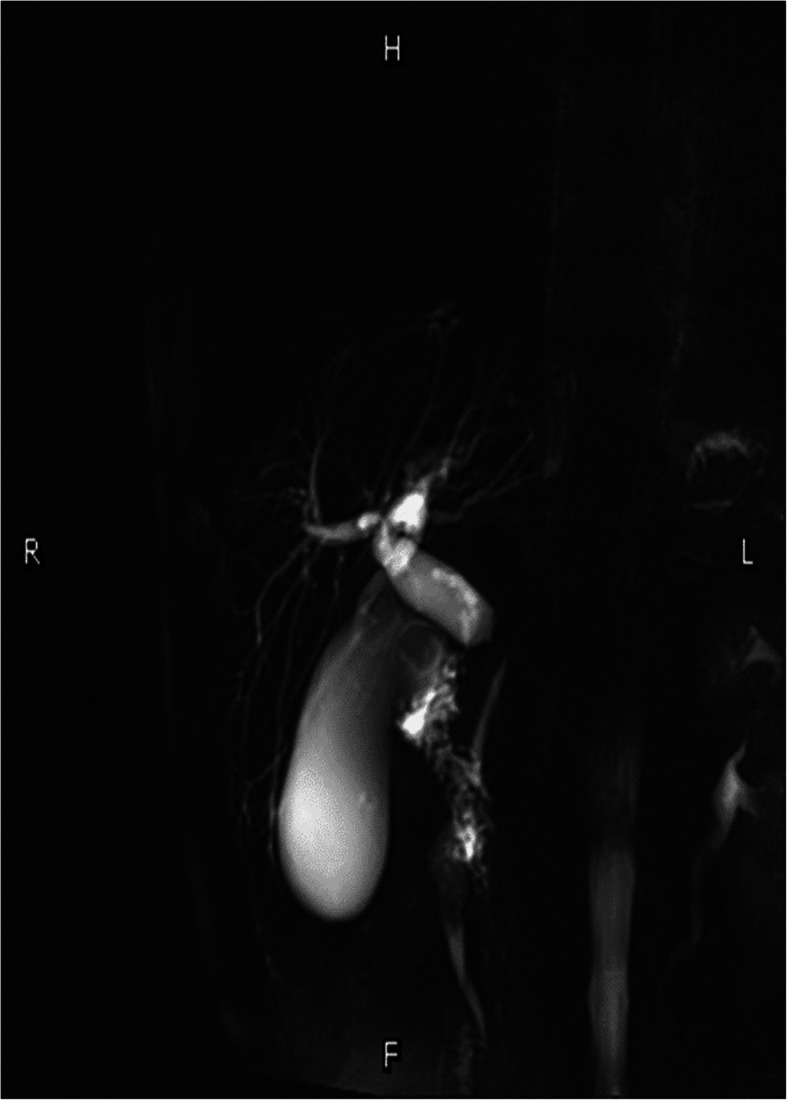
MRI showed local wall thickens and enhanced in the supper pancreatic segment of the common bile duct, and lumen is stenosis, mostly likely choledochal carcinoma, with upper bile duct dilatation
